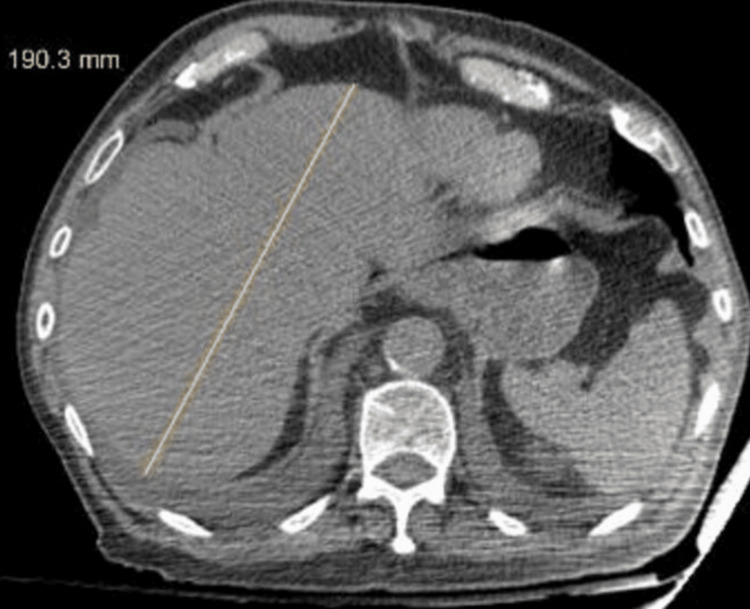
Abdominal CT demonstrating hepatomegaly (liver span approximately 19 cm) without focal hepatic lesions
